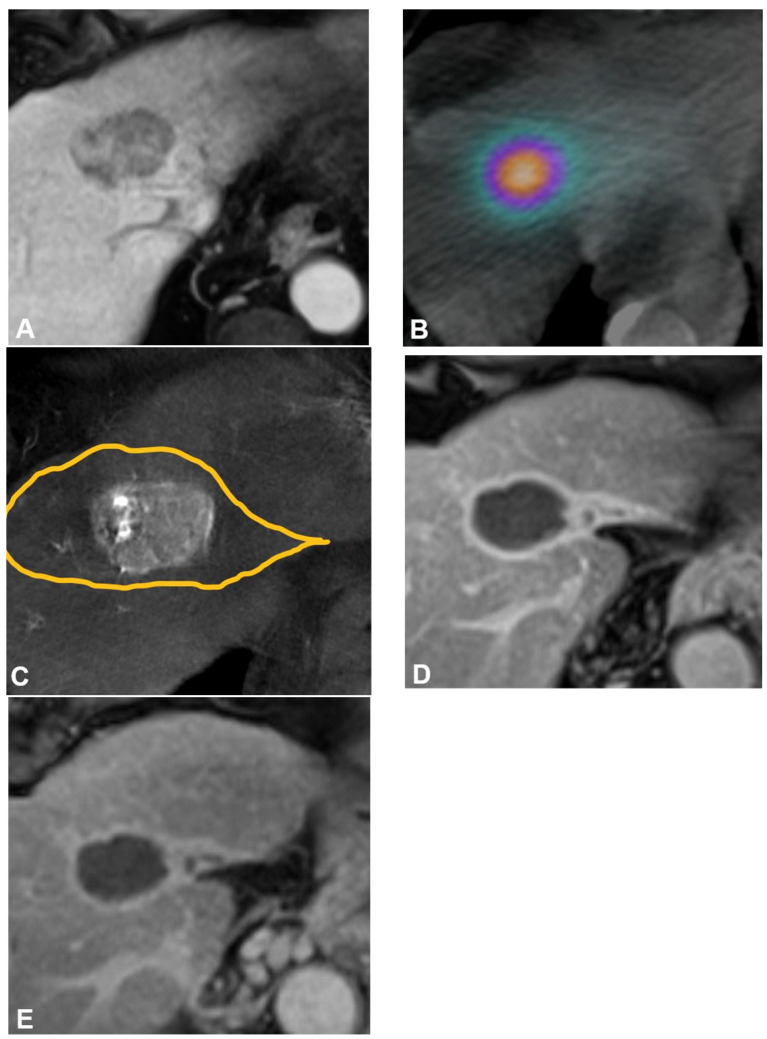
Radiation segmentectomy as sole therapy for hepatocellular carcinoma in a patient with a history of hepatitis C virus, stage 4 fibrosis, and ALBI 1 and Child-Pugh A5 liver function. (A) Pretreatment axial contrast-enhanced MRI demonstrates a 4.3 cm tumor in hepatic segment Iva. (B) Cone-beam CT performed at the time of mapping angiography demonstrates the arterially enhancing target tumor is fed by a segment IVa hepatic artery. The yellow line delineates the estimated treatment angiosome. (C) Posttreatment axial SPECT/CT confirms activity within the targeted angiosome and absent extrahepatic deposition. The estimated radiation dose was 870 Gy MIRD. Follow-up axial contrast-enhanced MRI obtained (D) 12 months and (E) 52 months after therapy demonstrate no evidence of residual or recurrent disease, and contraction of the treated angiosome.
